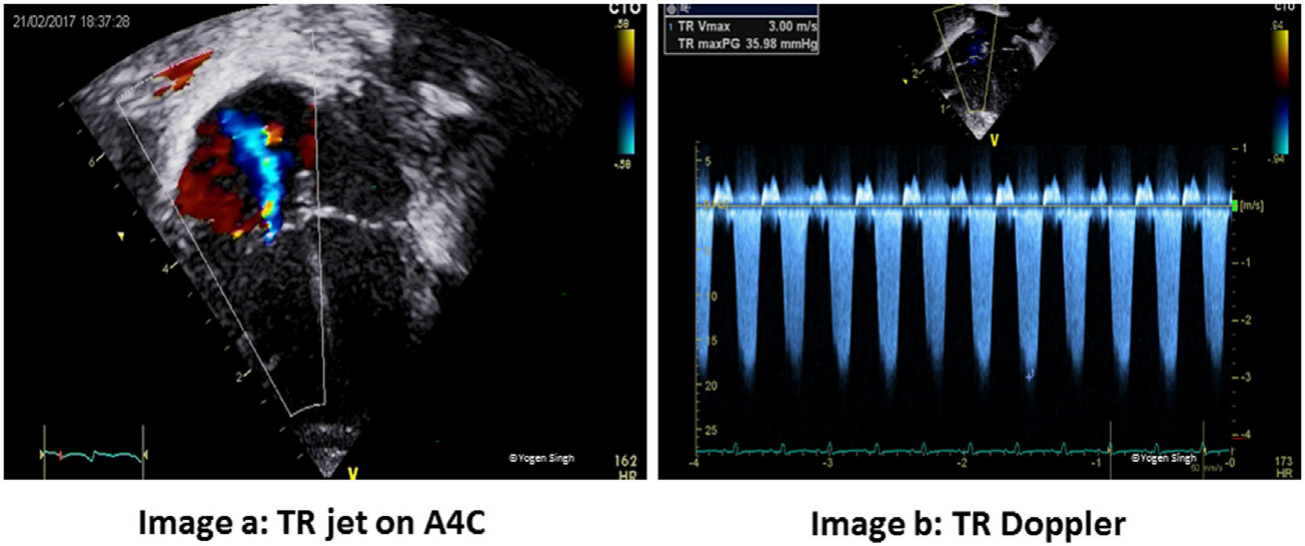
Quantitative assessment of pulmonary artery systolic pressure (PAP) by measuring tricuspid valve regurgitation velocity [tricuspid regurgitation (TR) jet]. PAP = right atrial (RA) pressure + pressure gradient between RA and RV (estimated by TR jet). (A) TR jet on A4C. (B) TR Doppler.
